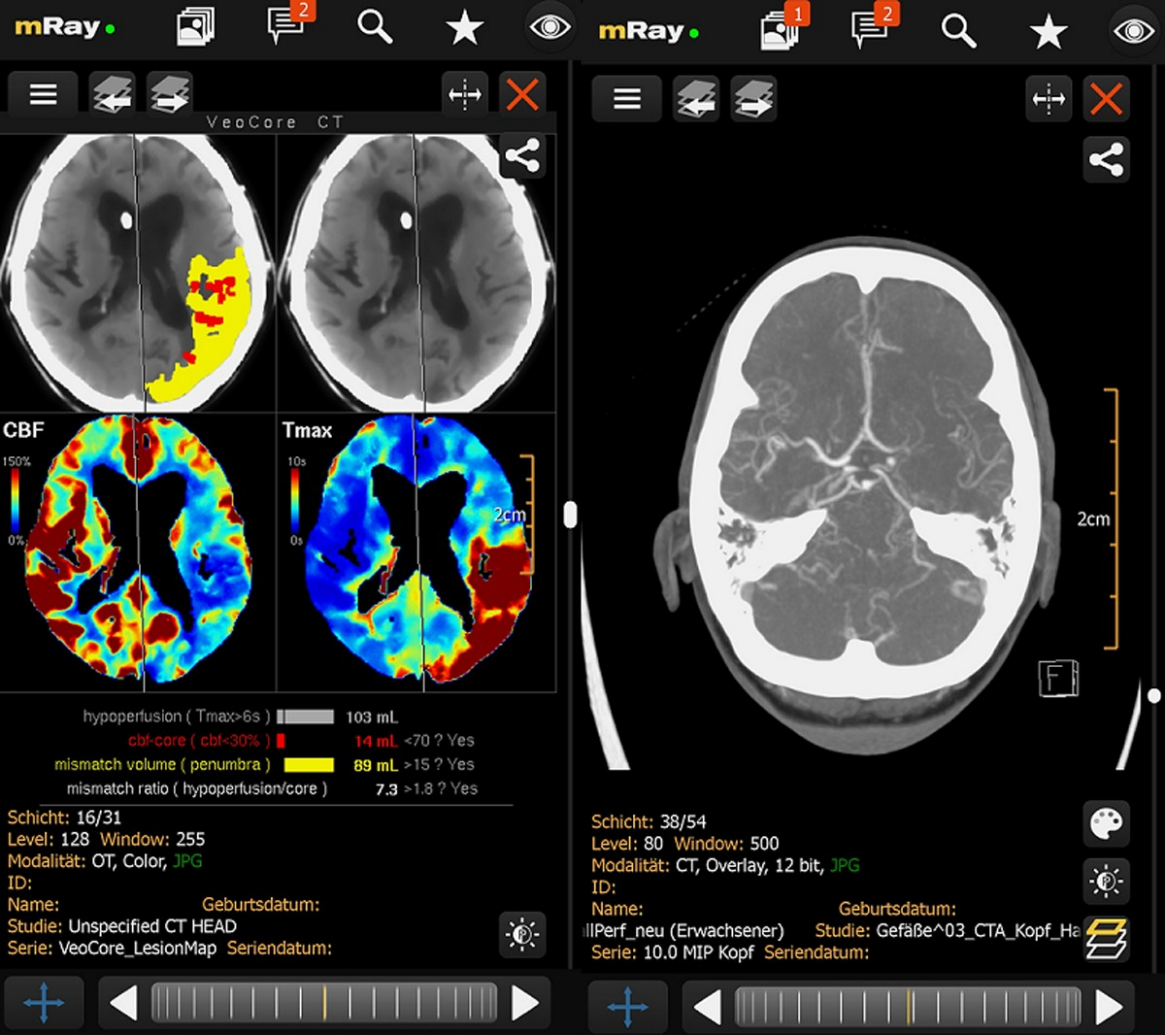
Veocore lesion map with core and mismatch and LVO on a CTA scan viewed with mRay.
